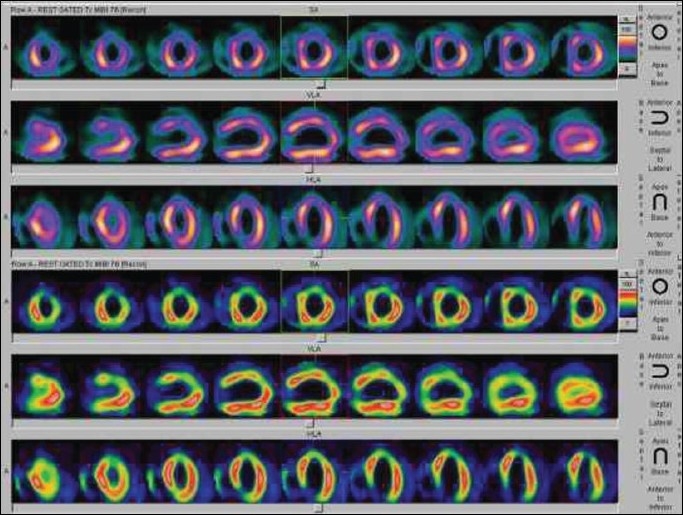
Rest gated MIBI SPECT images in a patient with dilated cardiomyopathy. Apparent perfusion defects seen in the apex and inferior segments of the LV myocardium
